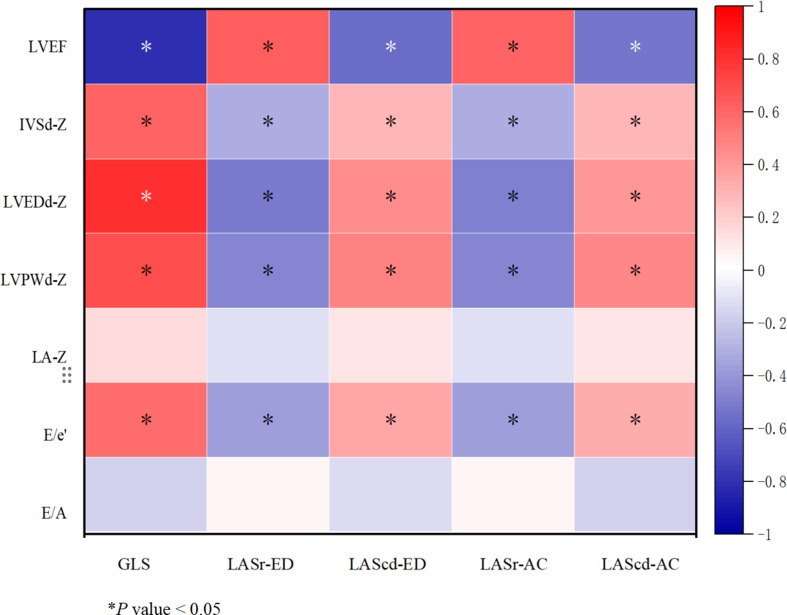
Correlation between left atrial strain and left ventricular function parameters of DCM patients. Red represents positive correlation, and blue represents negative correlation; the deeper and more saturated the color, the stronger the correlation between the two variables.P* value < 0.05. Abbreviations: GLS, Global longitudinal strain of the left ventricle; LASr-ED, longitudinal reservoir strain in end diastole; LAScd-ED, longitudinal conduit strain in end diastole; LASct-ED, longitudinal conduction strain in end diastole; LASr-AC, circumferential reservoir strain in peak systole; LAScd-AC, circumferential conduit strain in peak systole; LASct-AC, Circumferential conduction strain in peak; LVEF, left ventricular ejection fraction; TMAD AP4 Midpt%, mean displacement of the mitral annulus in the apical four-chamber view; e’, e’ of mitral valve pulse on doppler imaging; E/A, E peak- to-A peak ratio; and E/e’, E peak-to-e’ peak ratio
